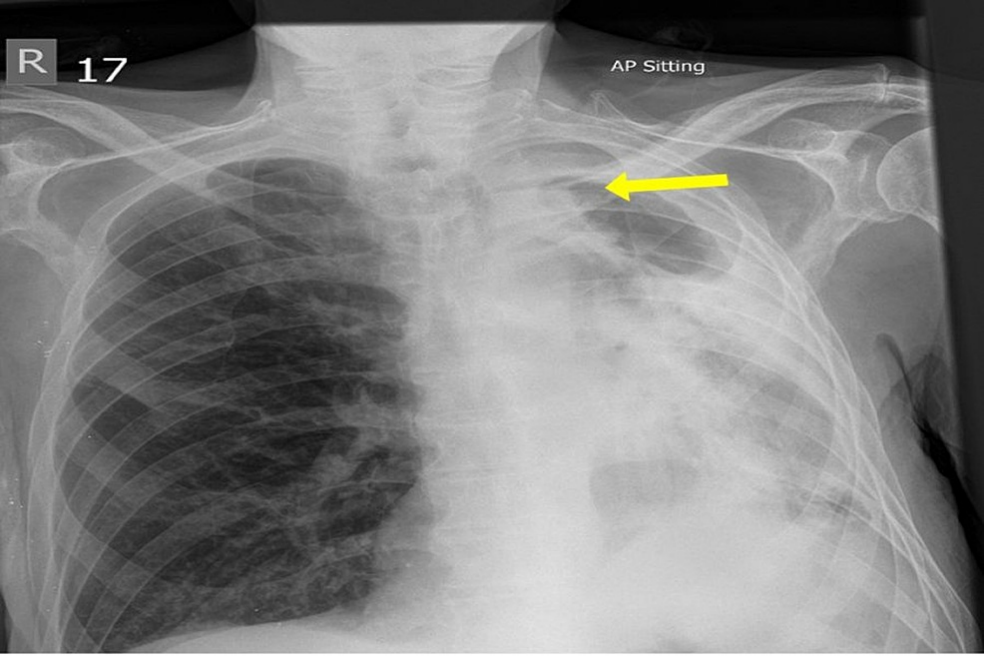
Chest X-ray: Antero-posterior view. There are long-standing changes, loss of left lung volume, left apical cavitation (yellow arrow), and extensive pleural thickening together with chronic fibrotic changes within the left upper lobe.
